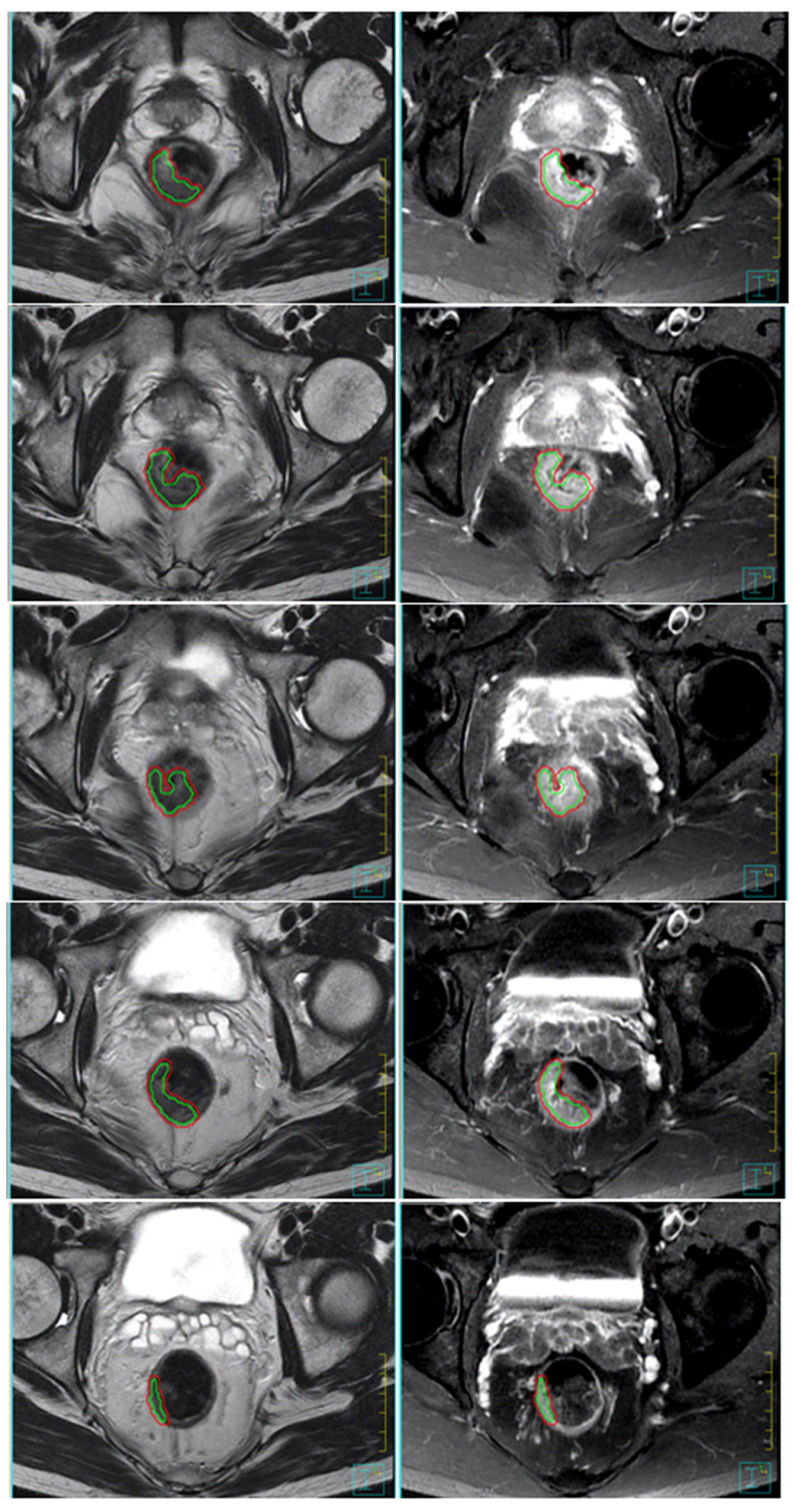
The rectal tumors of one patient are contoured as the ROI_RTV on the axial images (here, T2-weighted and contrast-enhanced T1-weighted MRI) for each tumor-containing lesion The red line encompasses the RTV area of every cross-sectional slice. The ROI_RTV_1 mm, the green line, was generated from the ROI_RTV with a 3-dimensional universal contraction of 1-mm length. The area between the outer red line and inner green line encompasses the outermost 1-mm layer volume as the surrogate of SA_RTV (RTV, real tumor volume; SA_RTV, surface area of RTV).
